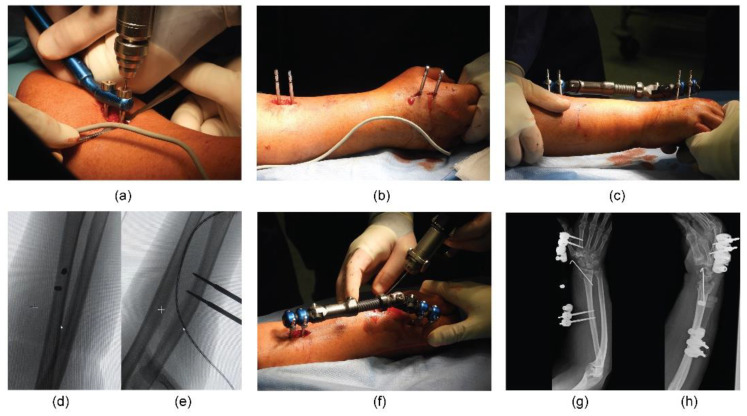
Intra-operative images showing: (a) insertion of the pins in the radial diaphysis between the middle third and the distal third of the forearm; (b) all pins positioned correctly; (c) final application of BEF; (d) antero-posterior (AP) and (e) latero-lateral (LL) fluoroscopic views of the proximal pins; (f) insertion of the KW at the radial styloid level. Post-operative radiographic images: (g) AP and (h) LL views showing proper reduction and stabilisation of the DRF (AO: 23-C2).
